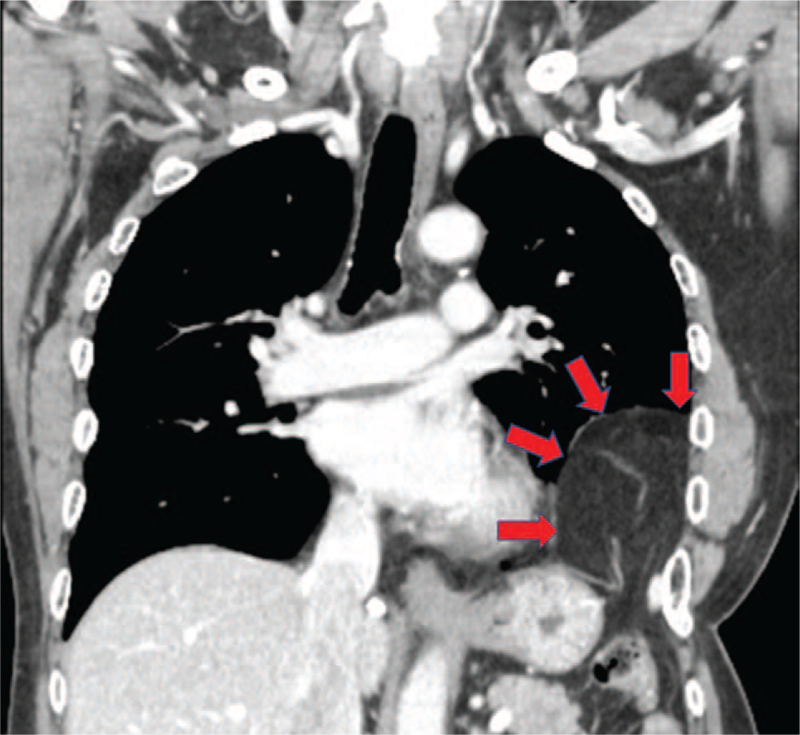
Chest computed tomography angiography (coronal section). The red arrow shows the content of the hernia, suspected to be the greater omentum.
